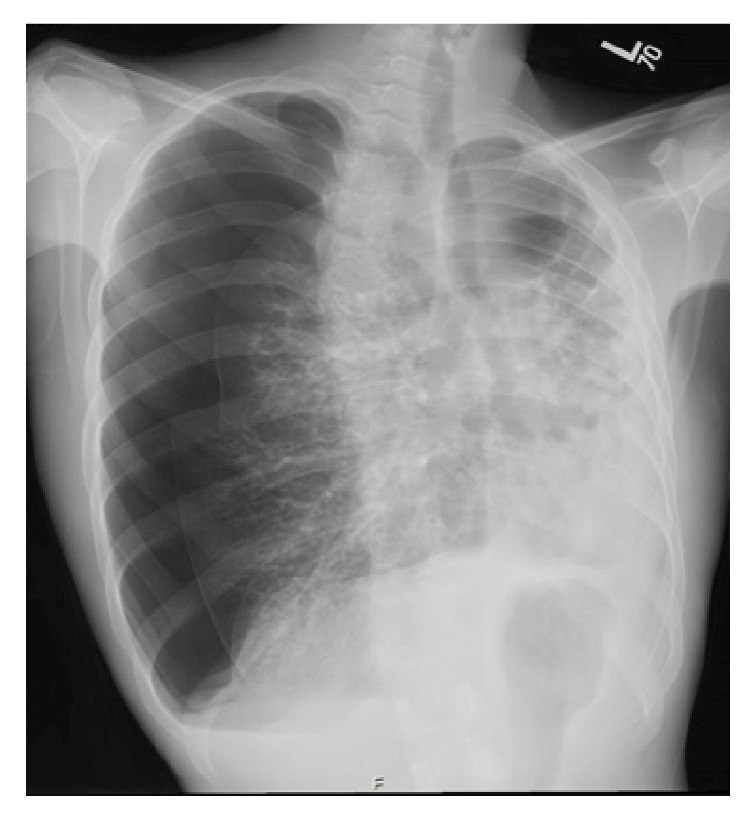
Chest X-ray on admission showing a right-sided pneumothorax prior to insertion of the chest tube.
